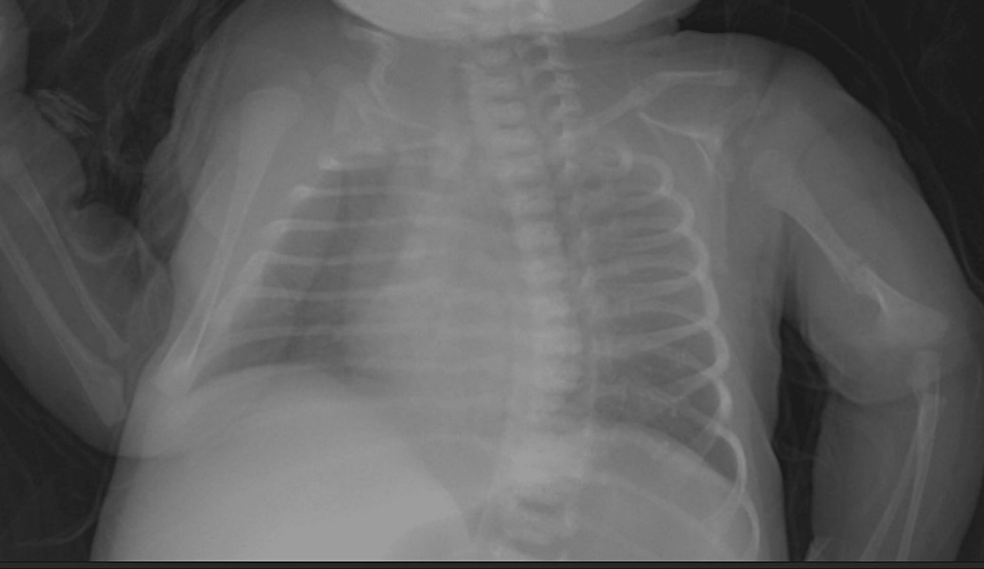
Radiography showing bilateral clavicle fracture and left humerus fracture
